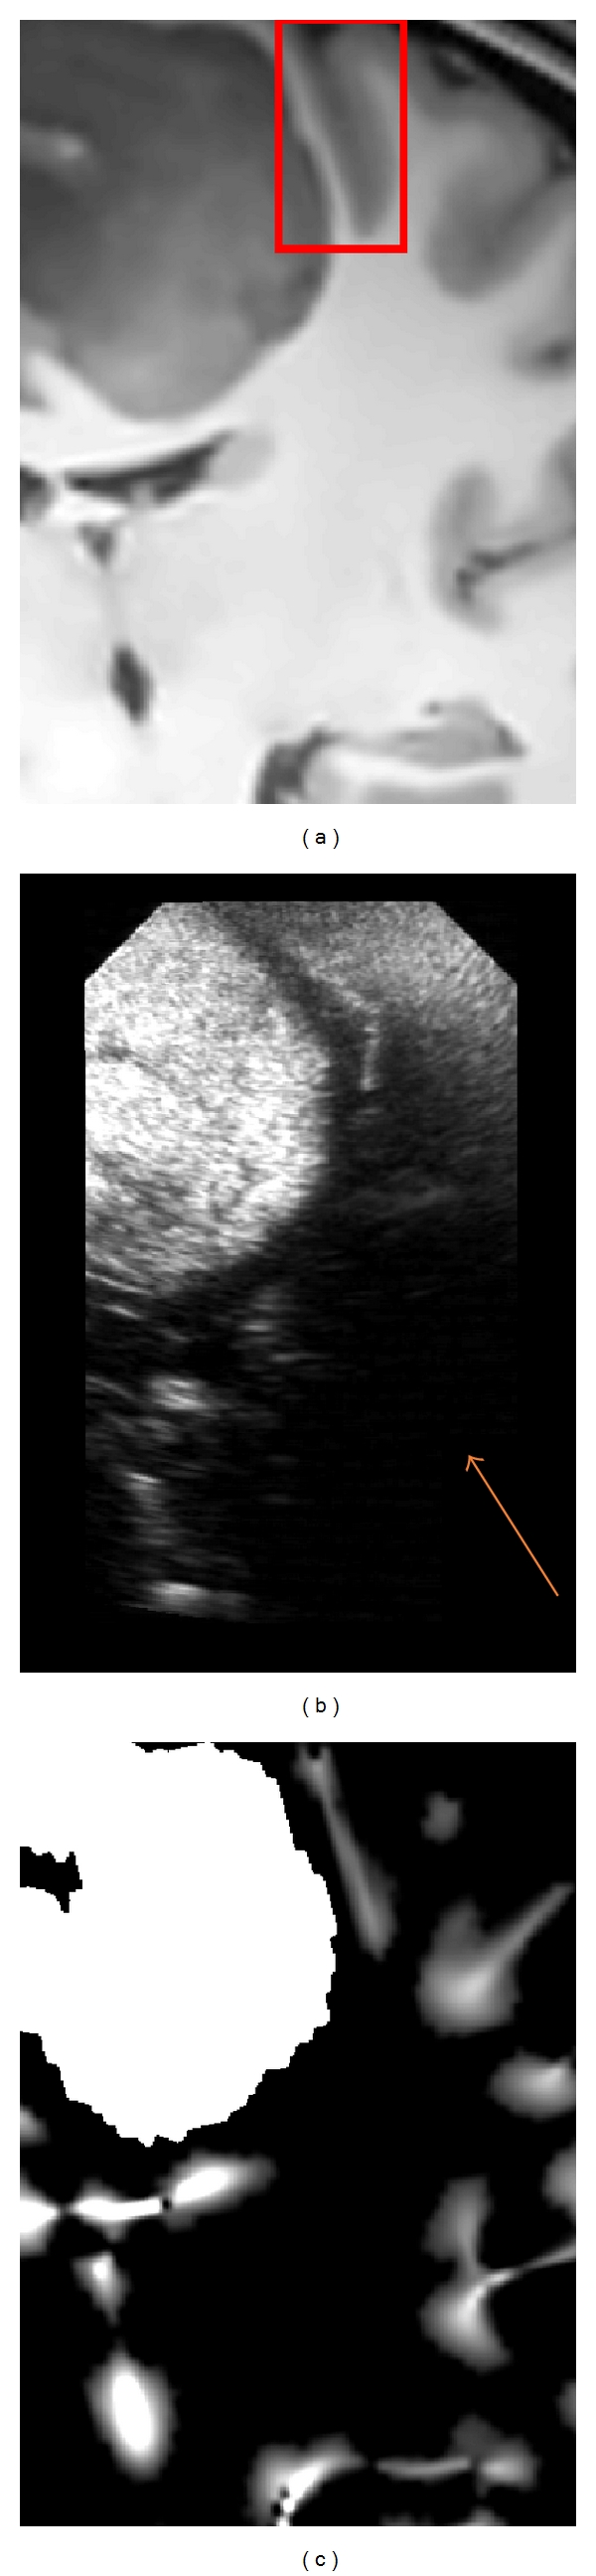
Patient 3. (a) The denoised MR image corresponding to US image (b). (c) The probability map based on *MLvv* operator and extracted from denoising MR image. The initial error is estimated around 5 mm by the experts for this patient. In the red box, the *MLvv* operator efficiently detects the sulci also visible in the US image. This case presents a limited field of view while the lesion is large. Moreover, an acoustic shadow is visible on the right lower part of the US image (indicated on the US image by a narrow).
